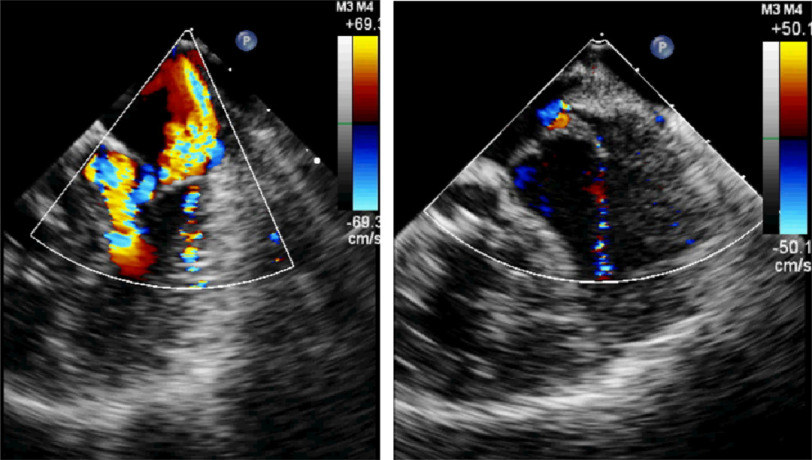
A. Pre-banding color flow mapping echocardiogram showing moderately severe, posteriorly directed mitral regurgitation. B. Post-banding image shows trivial residual mitral regurgitation.
